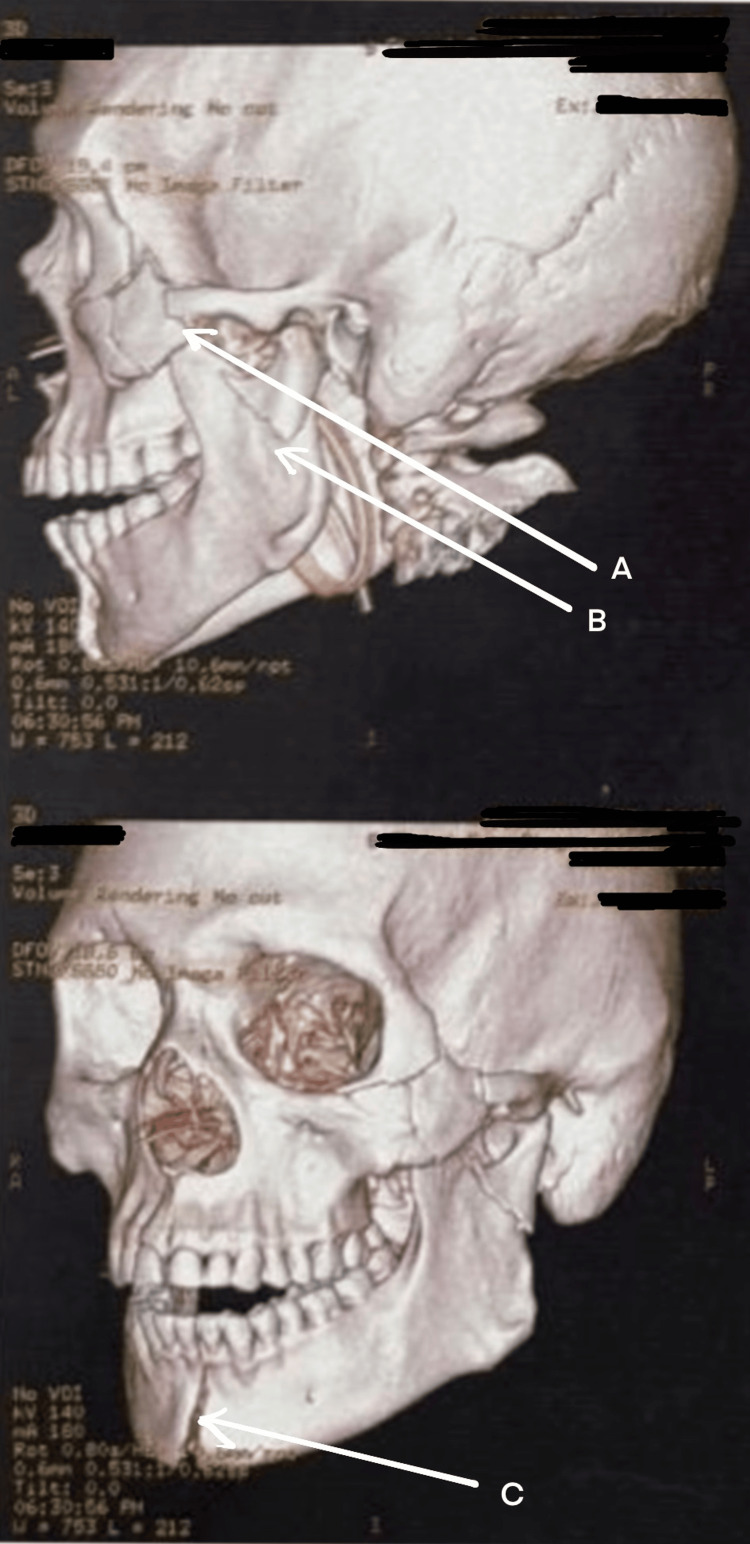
3D CT of the face and skull (Case 5) (A) Fracture of the left zygomaticomaxillary complex. (B) Fracture of the left condyle. (C) Fracture of the right mandibular parasymphysis.
